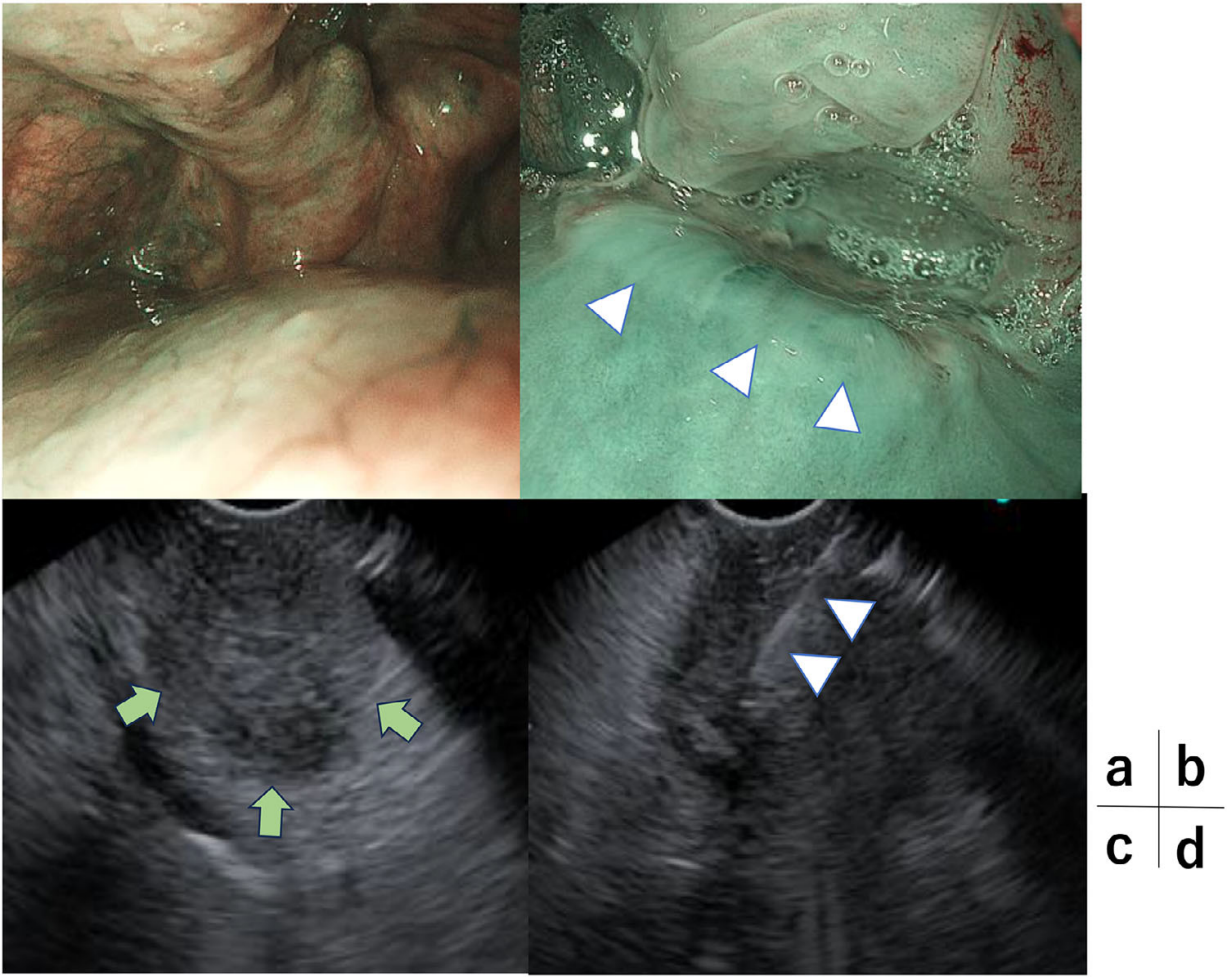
Endoscopic views. (a) Narrow‐band imaging (NBI) close‐up without laryngoscopic exposure (GIF‐H290T); the lesion is difficult to visualize. (b) NBI close‐up after laryngoscopic exposure (GIF‐XZ1200) showing a subepithelial‐like bulge with slight central depression on the posterior hypopharyngeal wall without mucosal exposure (arrowhead). (c) Endoscopic ultrasound (EUS) image (TGF‐UC260J) demonstrating a 22 × 18 mm, relatively well‐demarcated, ovoid hypoechoic subepithelial lesion (arrow). Internal echogenicity appears relatively homogeneous. (d) EUS‐fine needle biopsy (EUS‐FNB) image showing needle puncture with an Acquire S 22‐gauge needle under EUS guidance (arrowhead).
